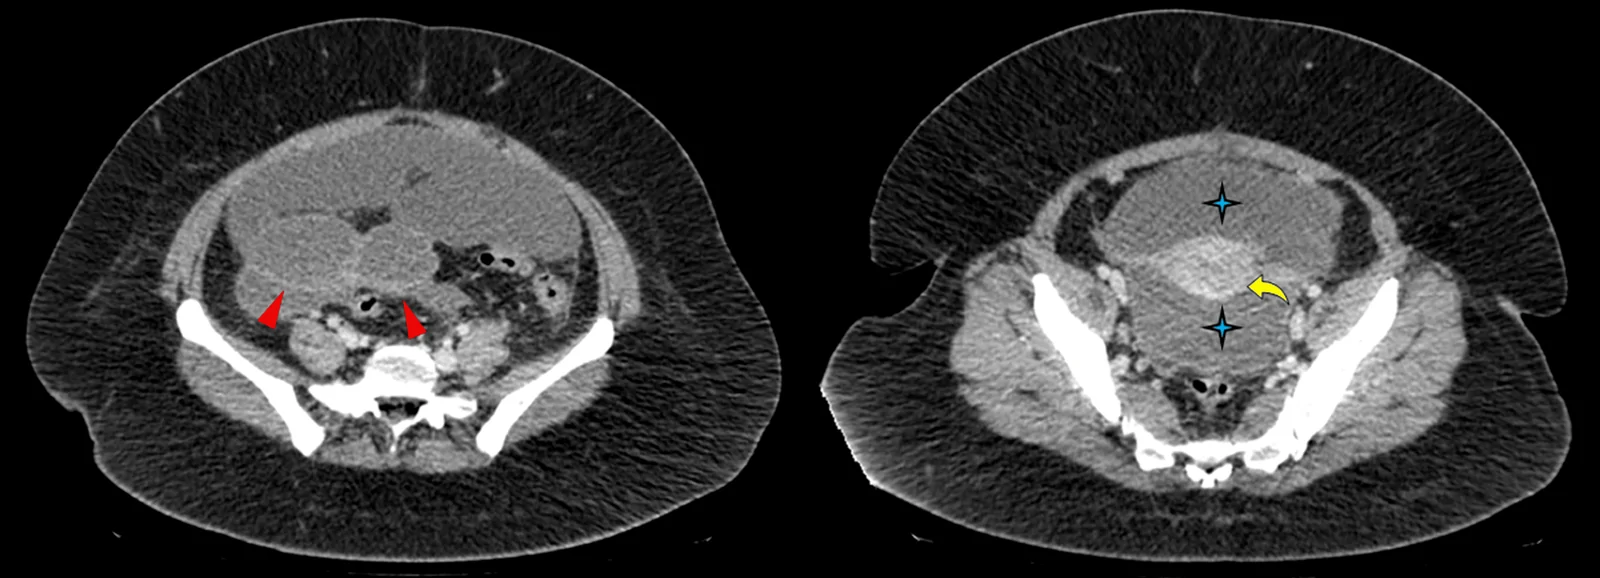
Abdominal CT scan (axial view) Right adnexal lesion with a cystic appearance, well-defined walls (red arrowheads) and fluid in the pelvic cavity (blue stars); uterus appears normal (yellow curved arrow). CT: computed tomography
